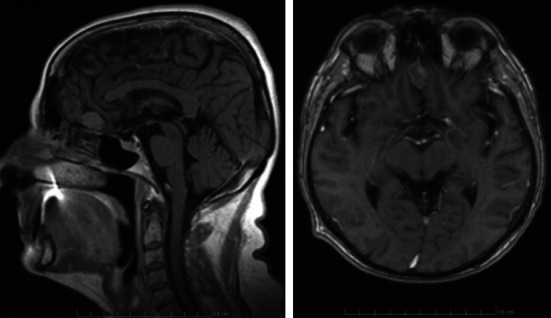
MRI (T2). Female patient aged 66—case number 3, history of anosmia for a year; vertigo, nausea and vomiting a week before admission
